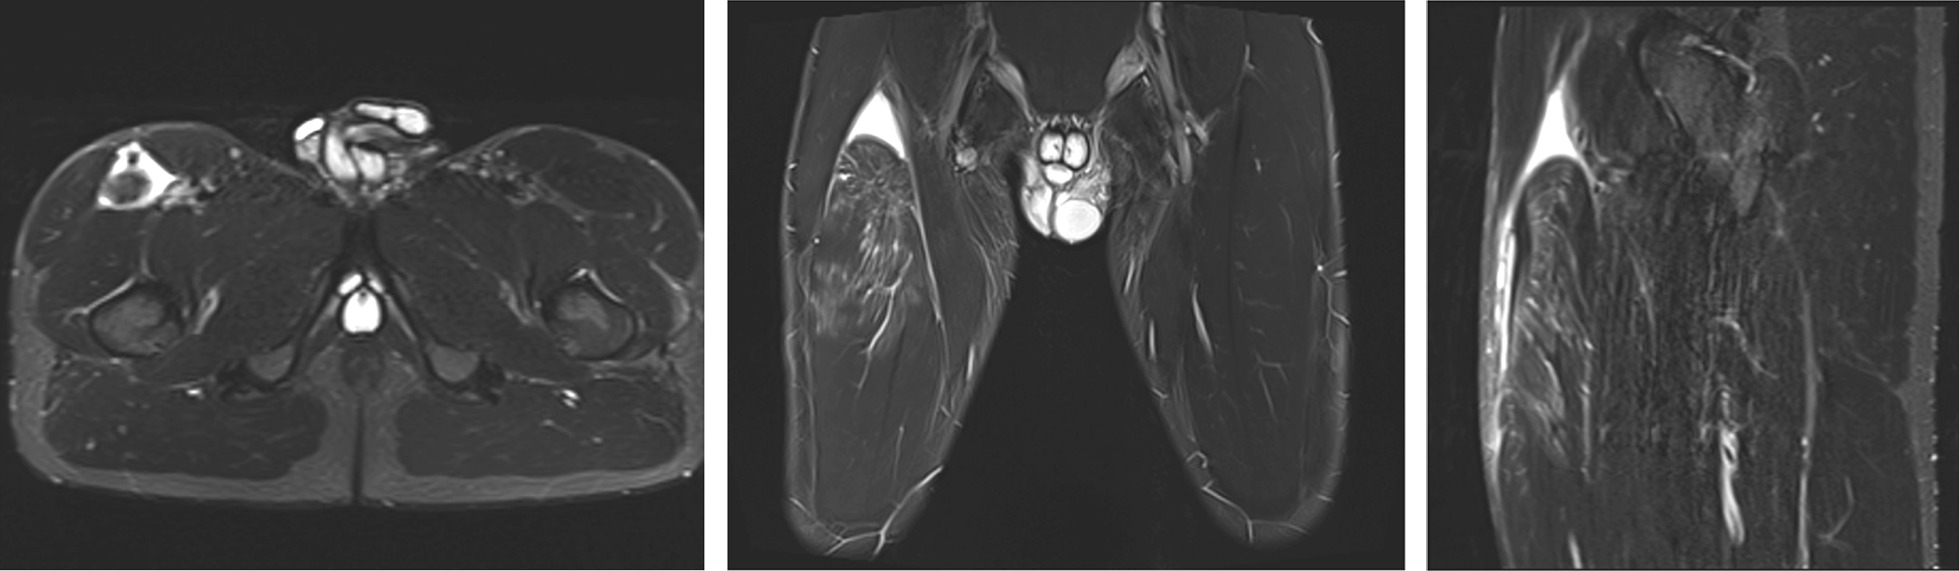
Coronal proton density fat saturated MRI image that shows a complete right proximal rectus femoris rupture at the conjoined tendon level with severe distal retraction of the muscle
